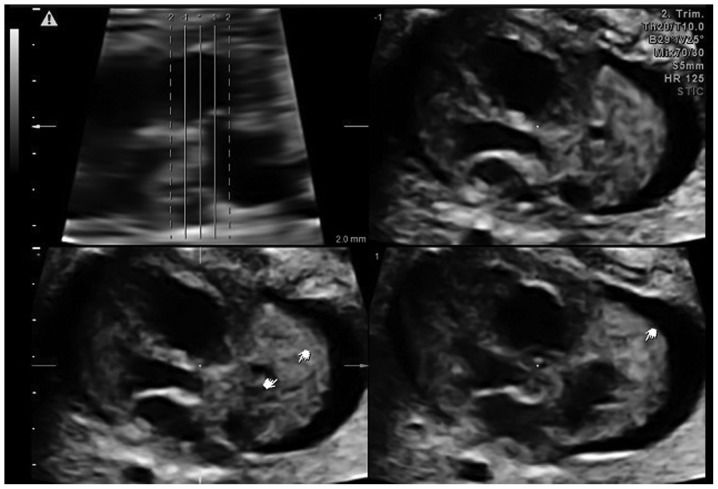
Tomographic ultrasound imaging results demonstrating a solitary tumor (indicated by pointers) located in the right atrium near the right atrial appendage (lower panels).
